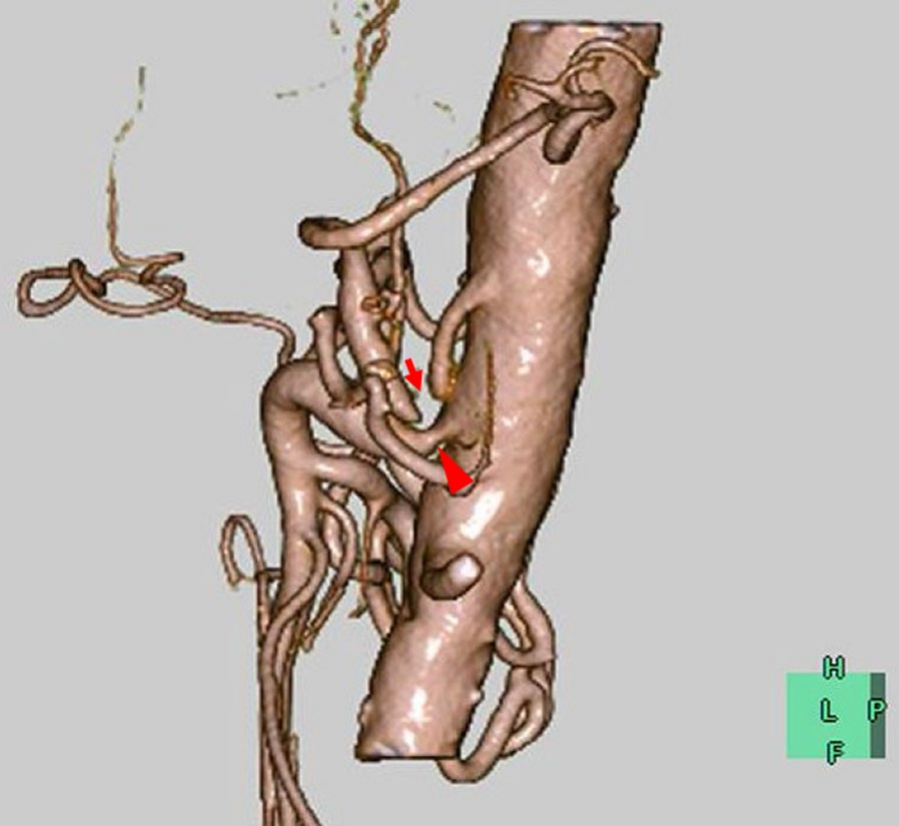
Three-dimensional reconstruction image of enhanced CT. Three-dimensional reconstructed image indicates an almost occluded celiac arterial root (red arrow) and mild stenosis at the root of the superior mesenteric artery (red arrowhead)
